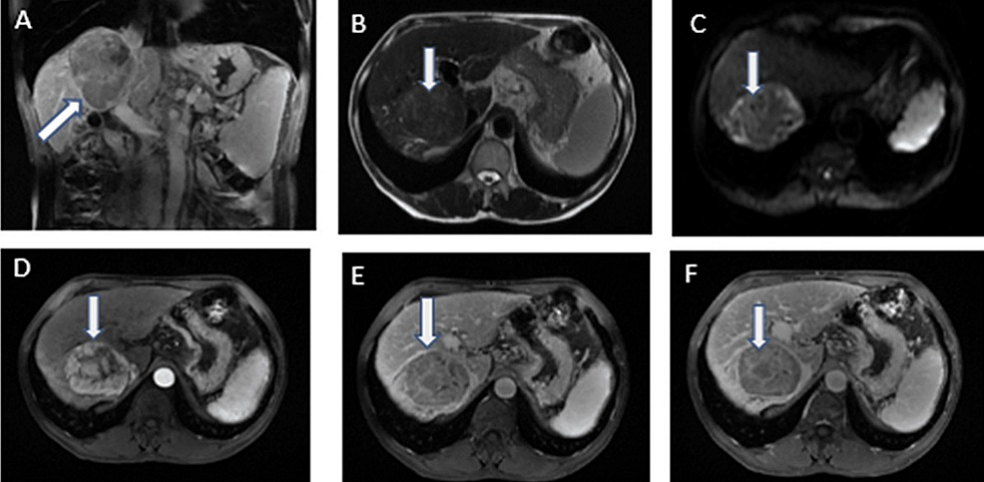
Hepatocellular carcinoma in a 66-year-old male. (A) Axial T1-weighted 3D AX GRE image shows hypointense lesion (arrow) in segment 8, (B) axial T2-weighted AX SSFSE BH image shows hyperintense lesion (arrow), (C) diffusion-weighted images show that the lesion remains hyperintense, (D) the lesion (arrow) is hypervascular on arterial phase with (E) washout on portal-venous phase, and (F) on hepatobiliary phase the nodule (arrow) is hypointense with surrounding liver parenchyma. 3D AX GRE: three-dimensional axial gradient; SSFSE BH: single-shot fast spin echo black blood
